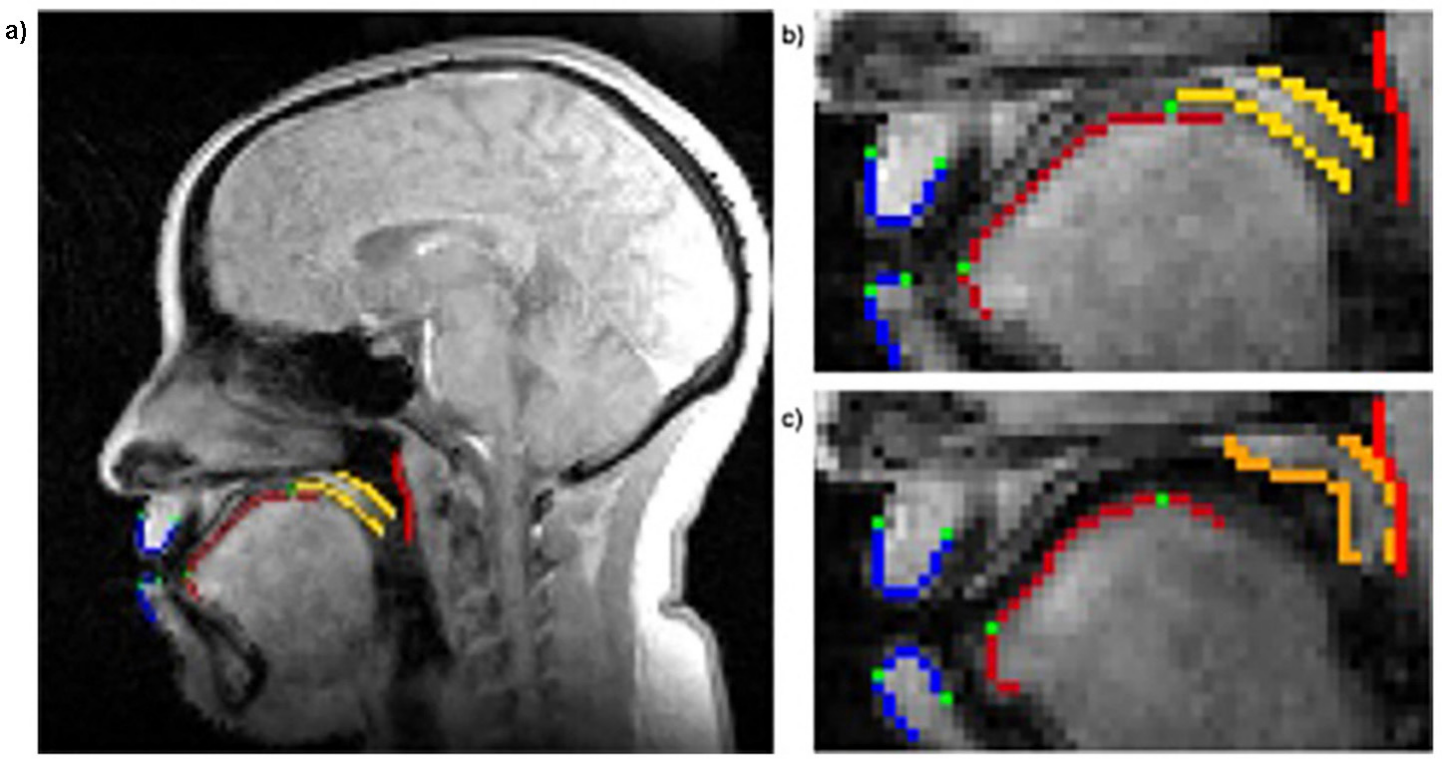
Articulator tracking outcome, with the green points representing the core coordinates used for statistical analysis: lower lip tip, lower lip wall, upper lip tip, upper lip wall, tongue tip, tongue dorsum. The posterior wall is tracked in red. (a) Mid-sagittal MRI image of the vocal tract showing traced contours of key articulators during speech. Velum interaction can be visualized when the velum pixels turn orange (c) from yellow (b).
